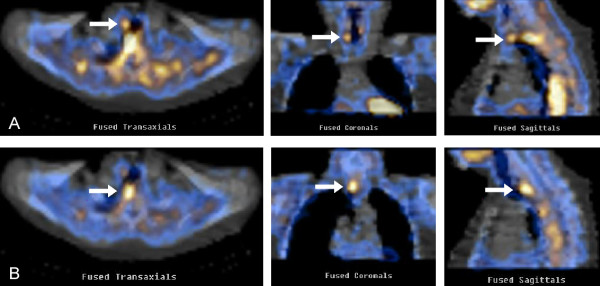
^99m^Tc-sestamibi scan with SPECT acquisition. Hyperfunctioning parathyroid glands (arrows) detected respectively to the right of (A) and behind the trachea (B).
